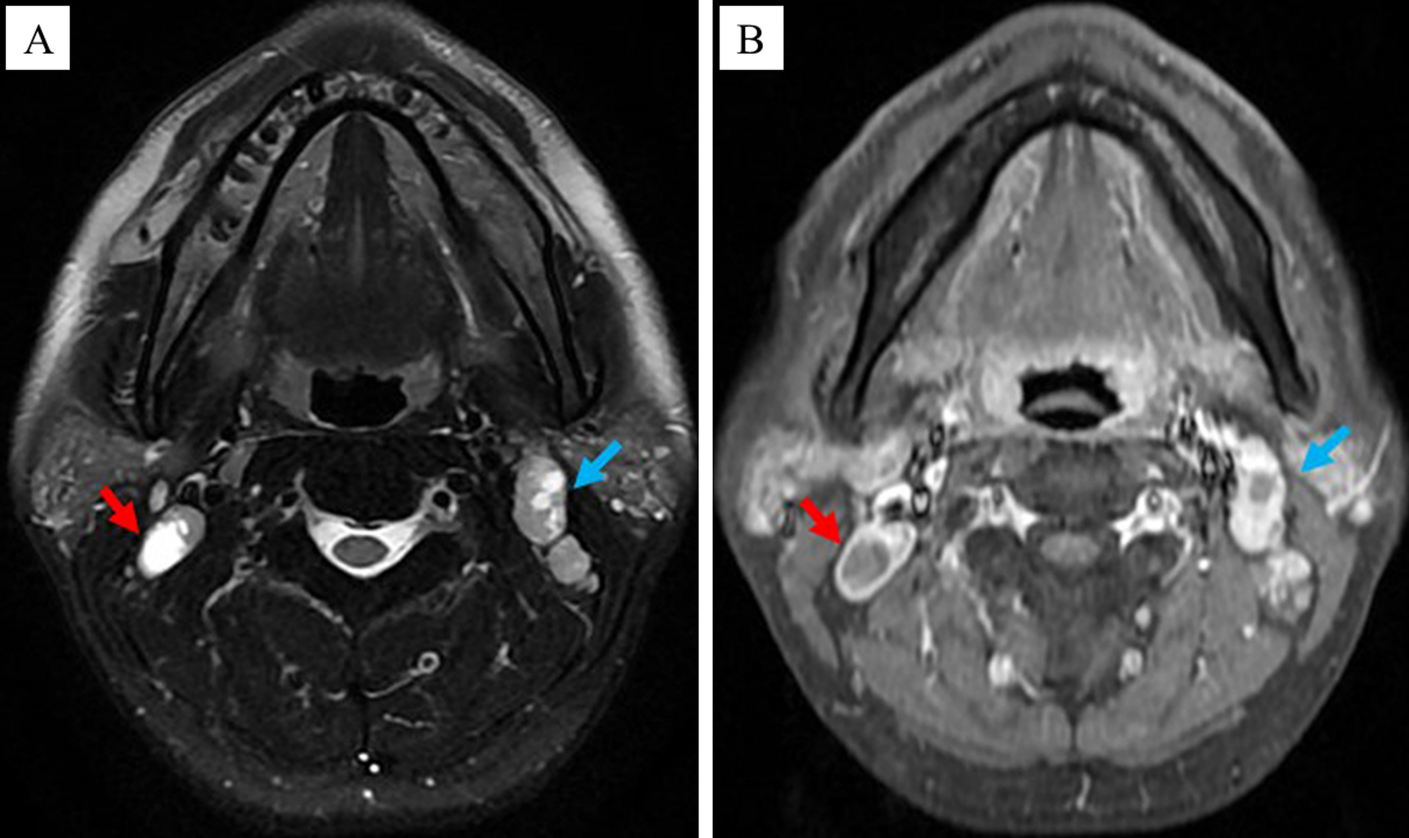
Principle of selecting the tCNN. If multiple CNNs were present in the same patient, the one with the largest proportion of necrotic area, as indicated by the red arrow rather than the green arrow, should be selected. (A) MRI images of the tCNN on the axial T2 sequence. (B) MRI images of the tCNN on the axial T1 enhancement sequence.
